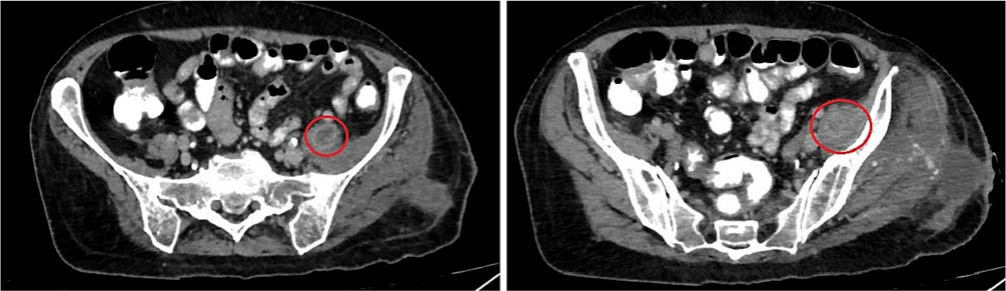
CT abdomen/pelvis showing rim enhancing fluid collection (marked with circle) in the left psoas muscle (left image) with extension to the left iliacus (right image) measuring 8.7 × 2 × 1.5 cm, suggestive of abscess formation.
